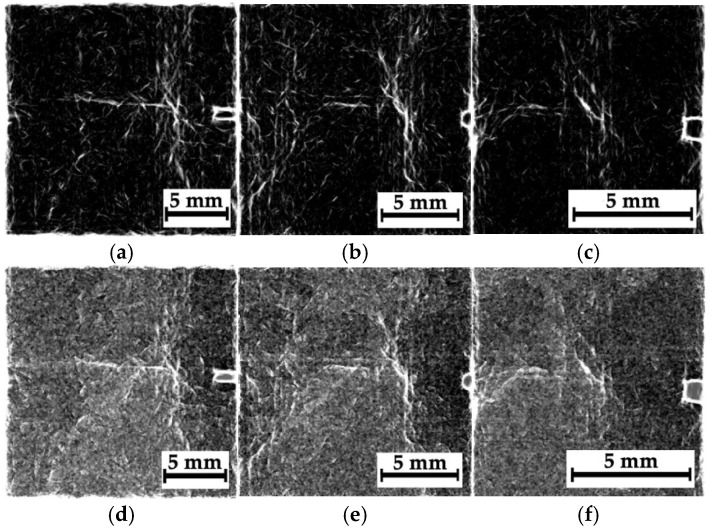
The results of processing the radiographic images, shown in Figure 13, as the phase variation images (a–c), as well as mixtures of both original images and phase variations (d–f); the distances between the sample and the sensor are 39 mm (a,d), 160 mm (b,e), and 240 mm (c,f).
